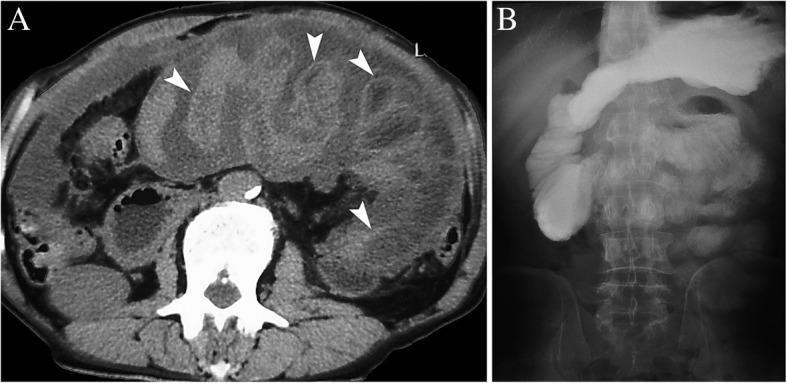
a: CT scan showed the small bowel wall thickening and membrane covering the small bowels (arrowhead), and the ascites has greatly diminished; b: Gastrografin meal follow through study revealed adherent small bowel loops with delayed transit time
